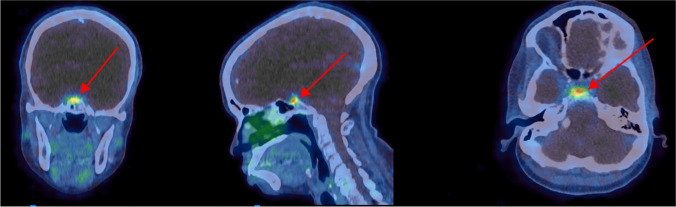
[68Ga]Ga-DOTA-TATE PET/CT of the head-and-neck region of a NET patient. Arrows indicate the radiopharmaceutical uptake in the pituitary region
